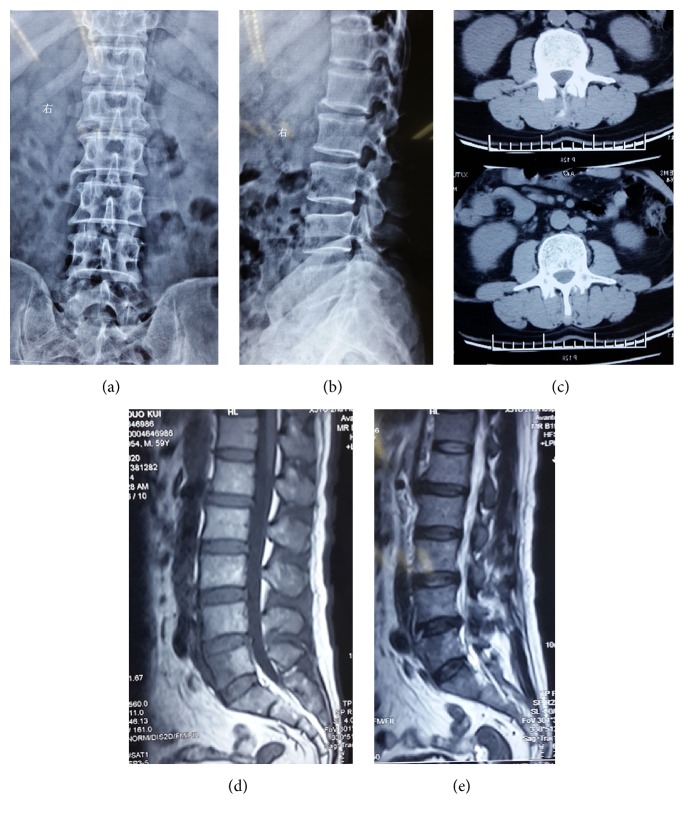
A 59-year-old male sustained fever and low back pain for 2 weeks. The anteroposterior and lateral plain film and lumbar CT scan were normal, but the MRI revealed a change in intensity at L5.
